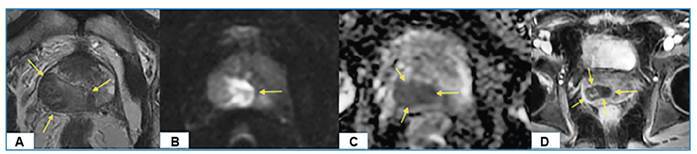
Multi-parametric prostate magnetic resonance imaging (MRI) findings. (A) T2-weighted image shows a heterogeneously hypointense lesion (yellow arrows) in the lateral-posterolateral section of the right peripheral gland. (B) Diffusion-weighted image demonstrates diffusion restriction within the lesion (yellow arrow). (C) The apparent diffusion coefficient (ADC) map reveals low ADC values corresponding to the lesion. (D) The post-contrast T1-weighted image depicts peripheral ring enhancement (yellow arrows) within the lesion.
